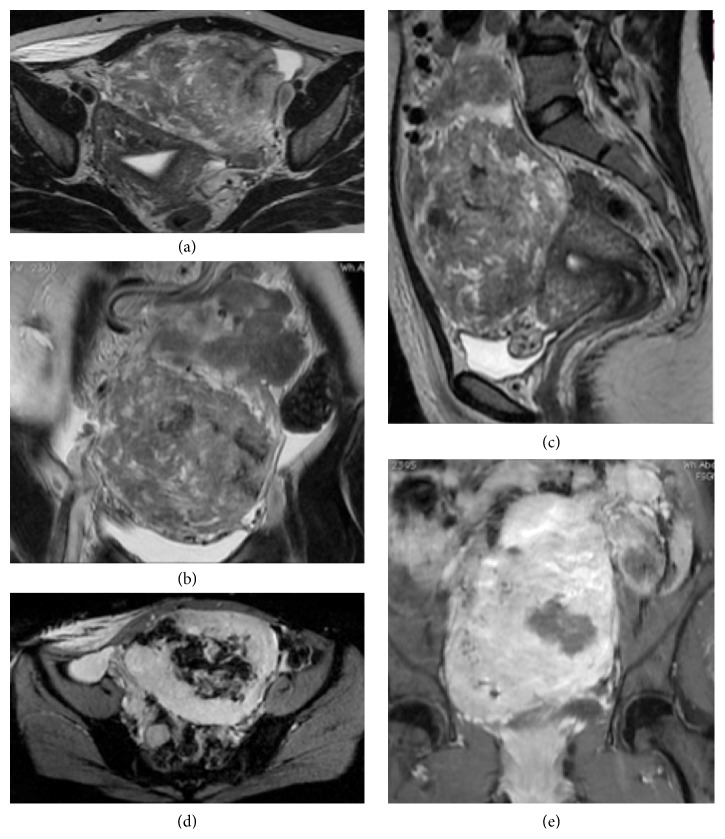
MRI of lower abdomen. Axial (a), coronal (b), and sagittal (c) sections of T2WI through the lower abdomen revealed a large heterogeneous pelvic-abdominal mass lesion in relation to the uterus. The mass appears to be inseparable from the adjacent mesentery and omentum. A tortuous vascular pedicle is also seen in the coronal section (b). Axial section of SWI sequence through the mass lesion showed areas of blooming suggestive of intralesional hemorrhagic foci (d). The mass lesion shows extensive enhancement after administration of gadolinium contrast (e).
